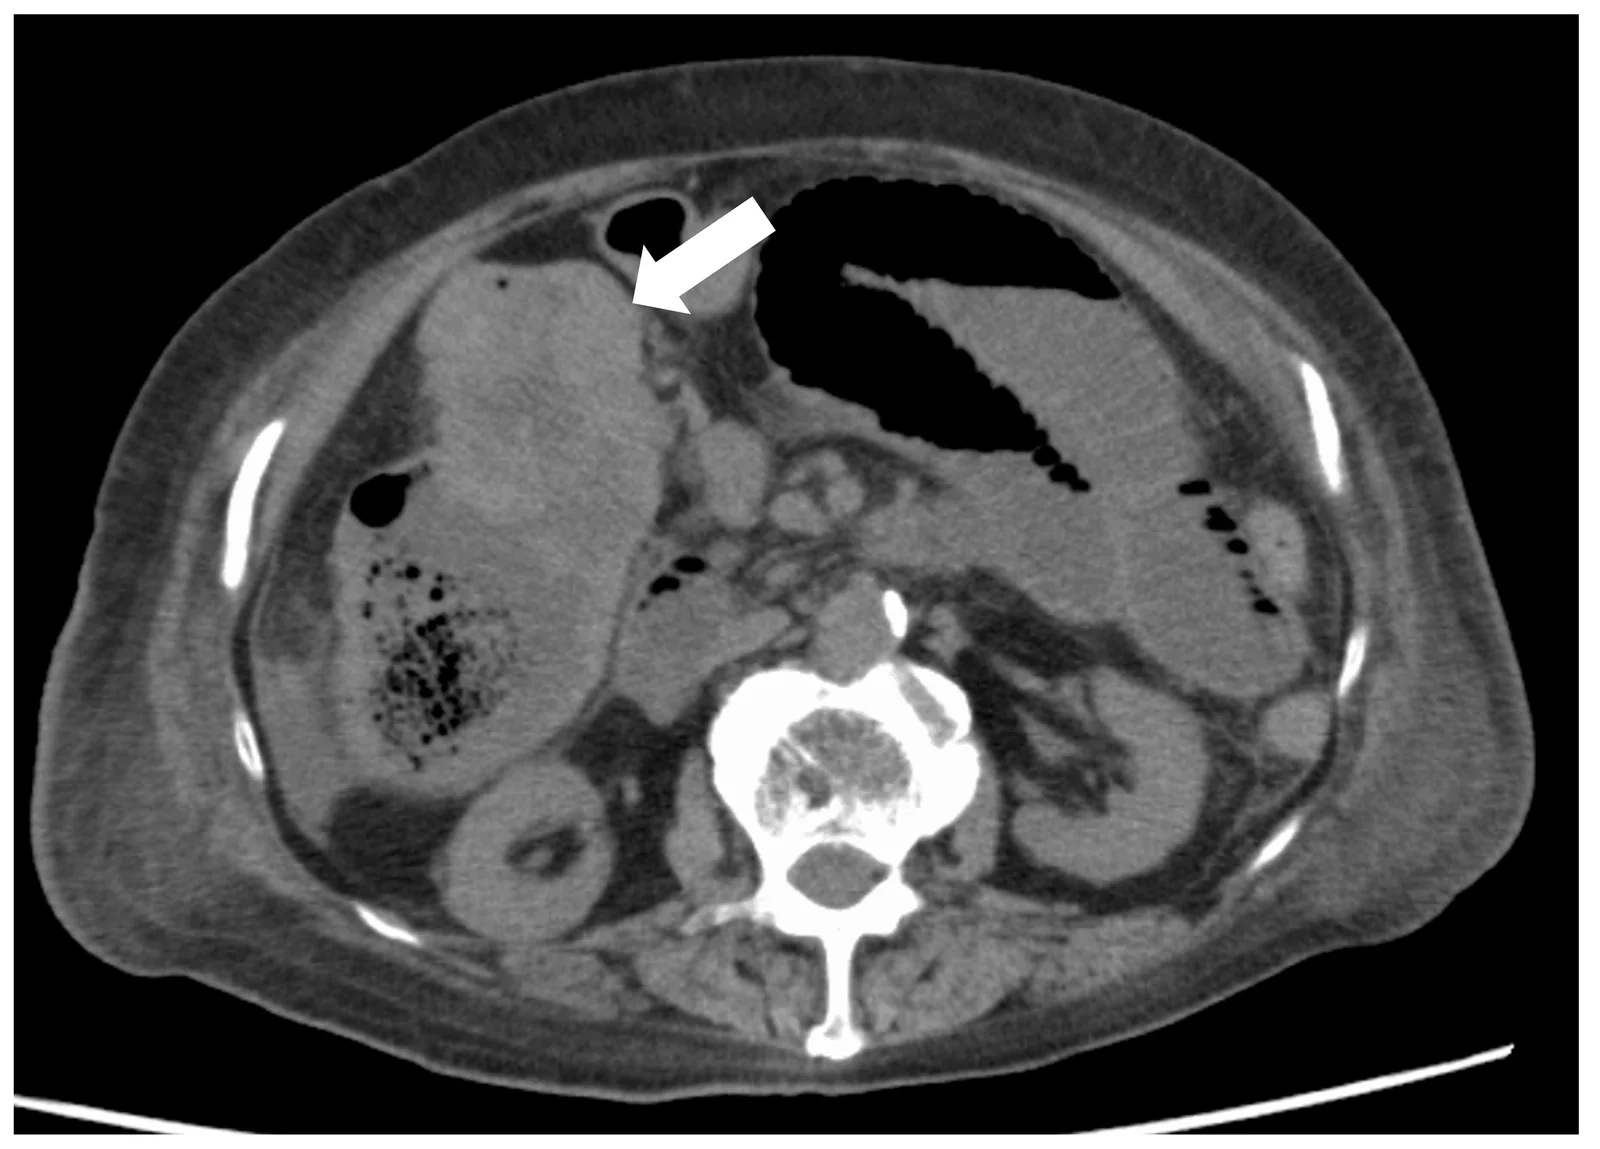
Unenhanced axial computed tomography image of the abdomen obtained on admission. Unenhanced axial computed tomography (CT) image of the abdomen obtained on admission, showing a circumferential mass (arrow) at the hepatic flexure of the transverse colon with marked mural thickening and irregular intraluminal morphology. The lesion caused near-complete large bowel obstruction with pronounced dilatation of the proximal colon. Contrast-enhanced CT was withheld because of underlying chronic kidney disease. No extraluminal gas or free fluid was identified, excluding perforation.
